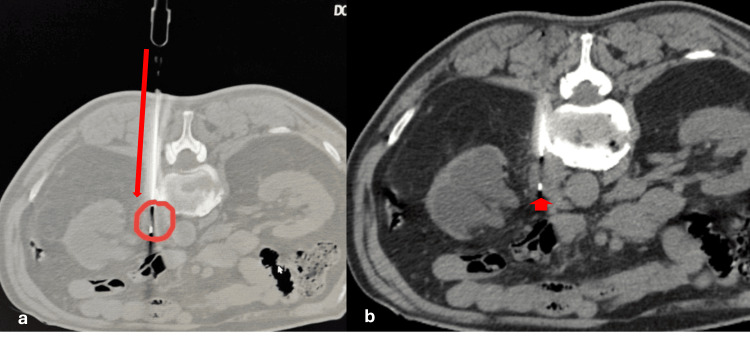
(a-b) Axial images of the access route during CT-guided coaxial core-needle biopsy of the left para-aortic mass. Note the needle’s access path (arrow) and the tip of the core needle within the lesion (circle in 2a and arrowhead in 2b), verified just before sampling.
